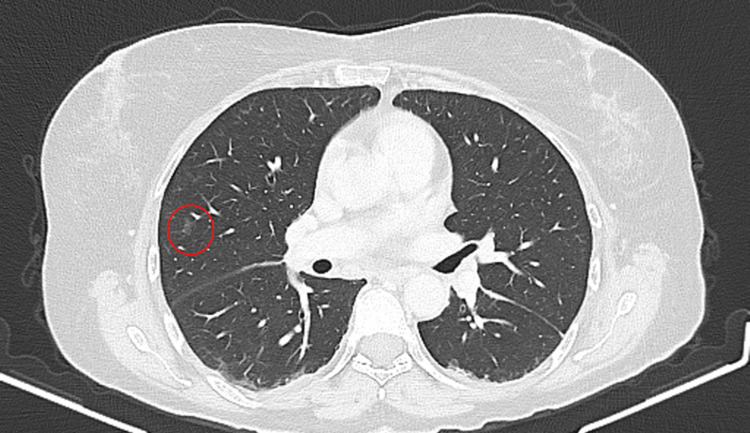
Chest CT 10 days after pembrolizumab administration An 8 mm ground-glass nodule in the right upper lobe (circle). CT: computed tomography
